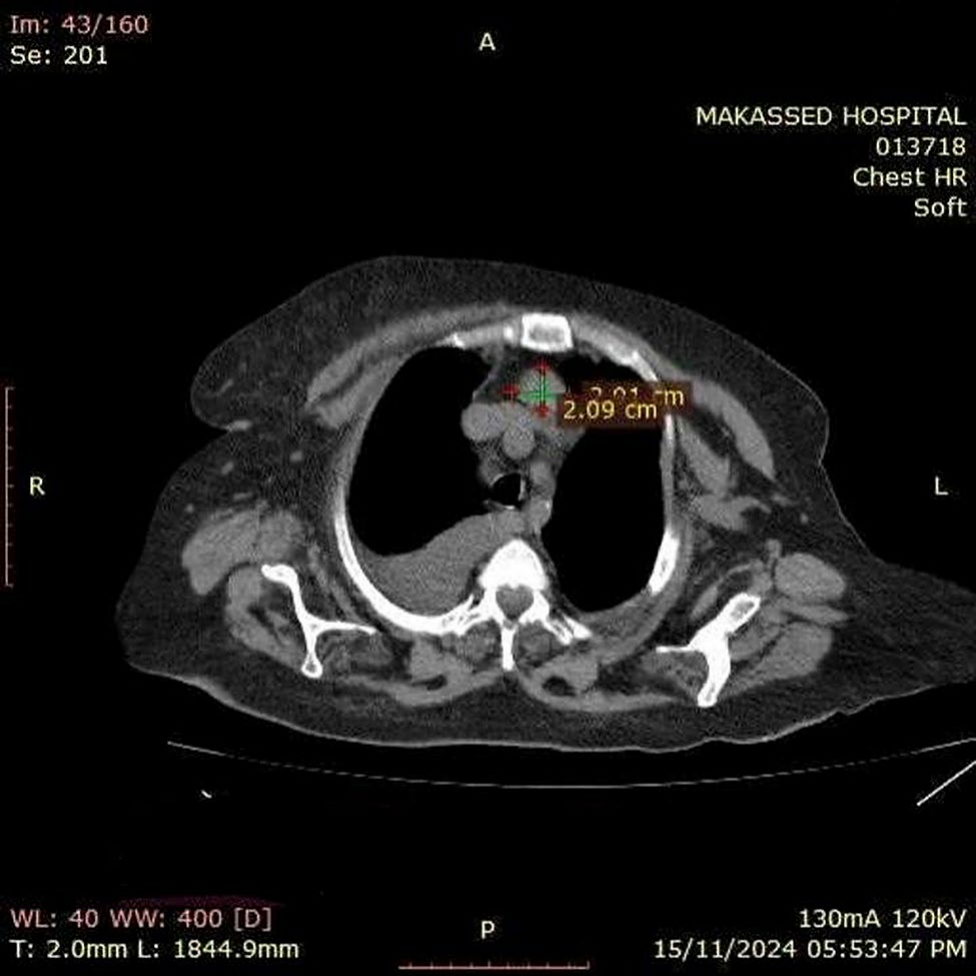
Chest CT scan showing a well-defined anterior superior mediastinal soft tissue mass measuring approximately 2.09 × 2.11 cm, consistent with ectopic parathyroid adenoma as indicated by parathyroid scintigraphy findings
